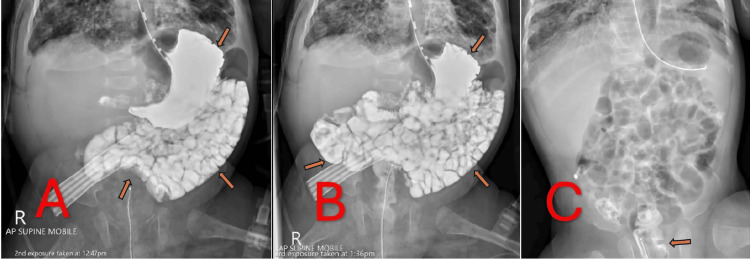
Upper GI Contrast Study Demonstrating Progressive Contrast Transit and Final Resolution A: Mid-phase image showing contrast passing from the stomach into the proximal small bowel, without any leakage; B: Later-phase image with further contrast transit into the mid-small bowel, confirming no extravasation; C: Final radiograph with residual contrast visible in the rectum, proving no perforation and confirming intact bowel continuity, alongside complete resolution of pneumoperitoneum.
